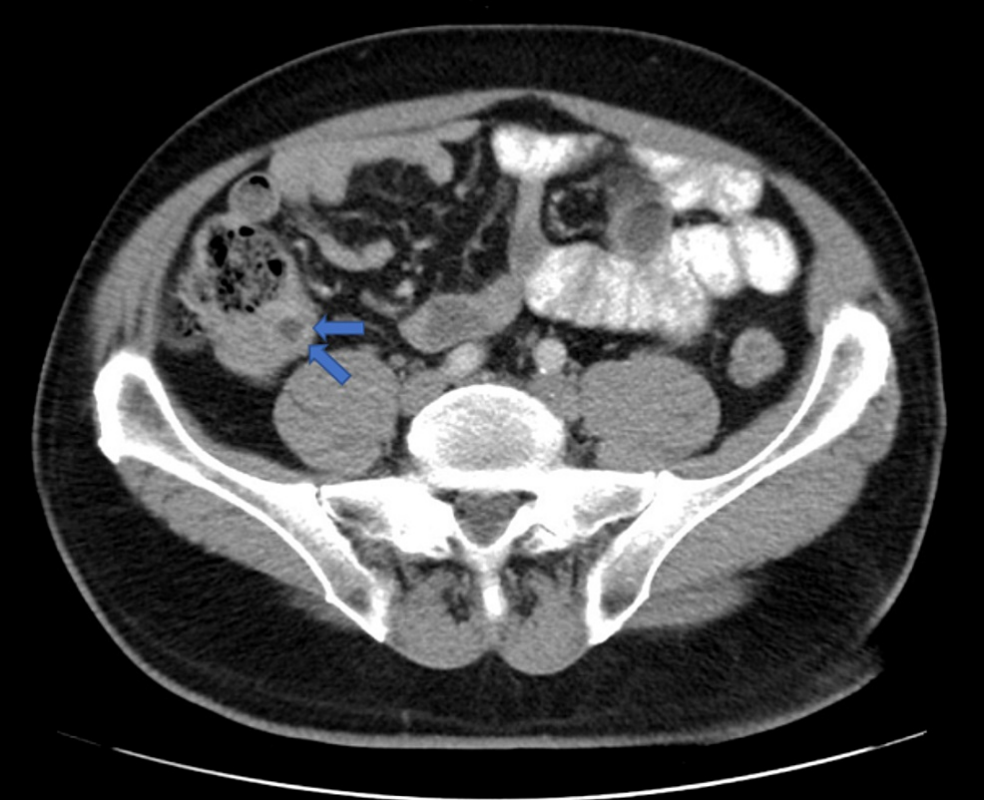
Abdominal transverse view CT scan with contrast demonstrating focal hypodense mass within the base of the appendix, which measures 1cm x 1cm. The more distal portions of the appendix are unremarkable. There is no evidence of periappendiceal fat stranding. CT: Computerized tomography
